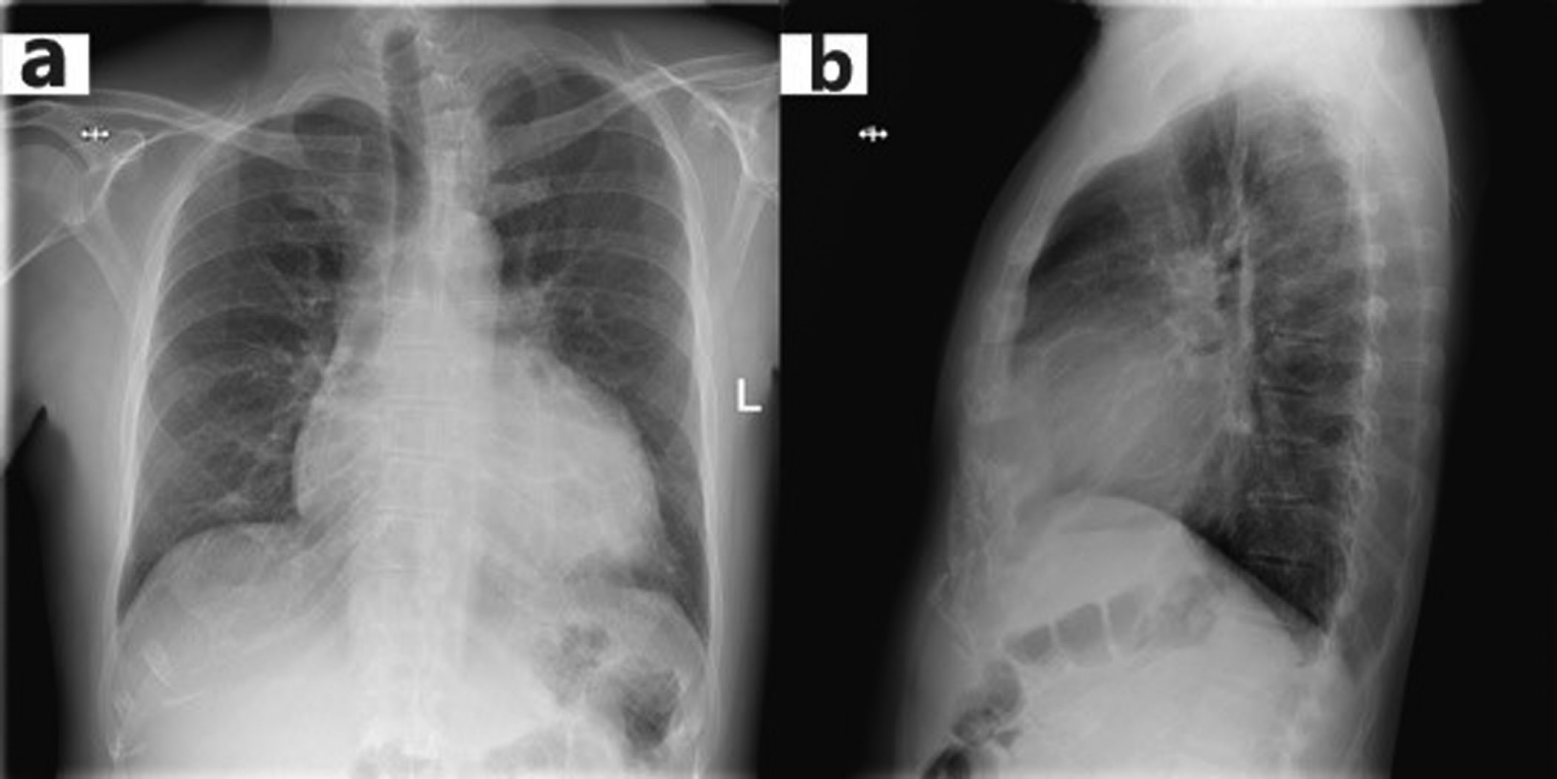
The chest X-Ray revealing cardiomegaly and increased cardiothoracic ratio (CTR). a: Face; b: Profile.
